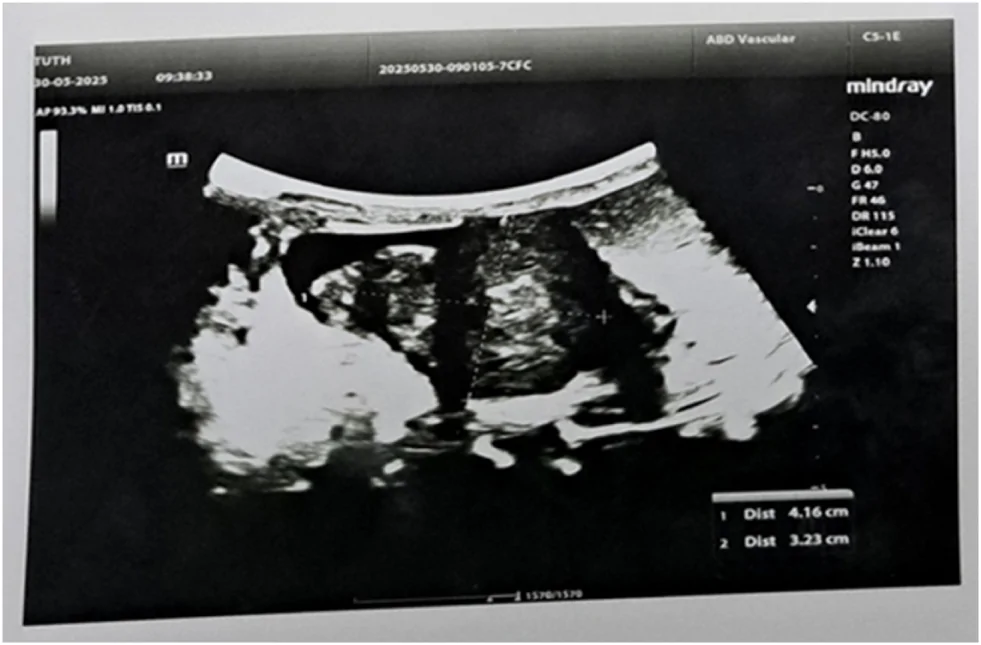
Scrotal ultrasonography revealed multiple hypo- to heteroechoic lesions in the right scrotal sac without internal vascularity.
